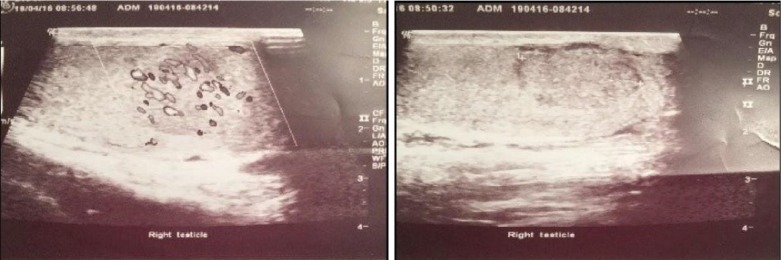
Scrotal ultrasound: right testicle.
